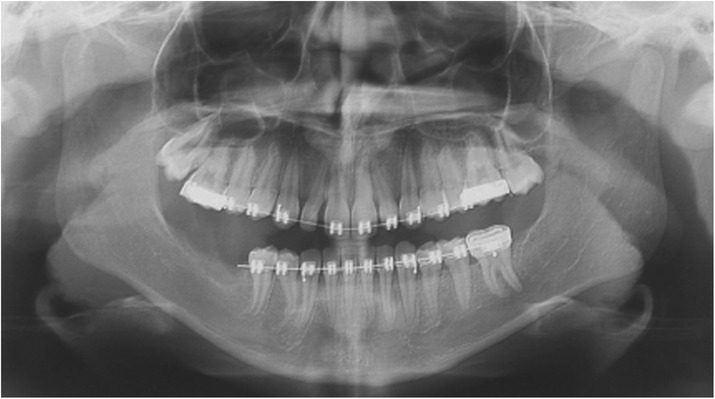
TA, female, diagnosis at age 9 – Follow-up at 5 years *(16-avr-2013)*. Panoramic radiography shows the good aspect of the bone mandibular structures and the absence of relapse
